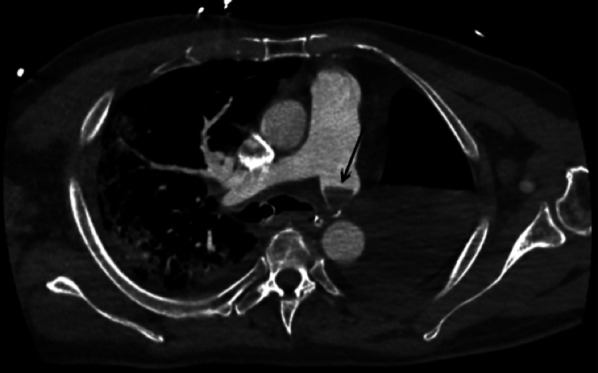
Alluvial thrombus. Axial CT image (mediastinal window) showing an alluvial thrombus in the left pulmonary arterial stump (black arrow)An interposition flap may cover the bronchial stump and show fatty attenuation.Finally, normal evolution of the post-pneumonectomy space can be towards complete resorption or the persistence of a residual pouch.D.
